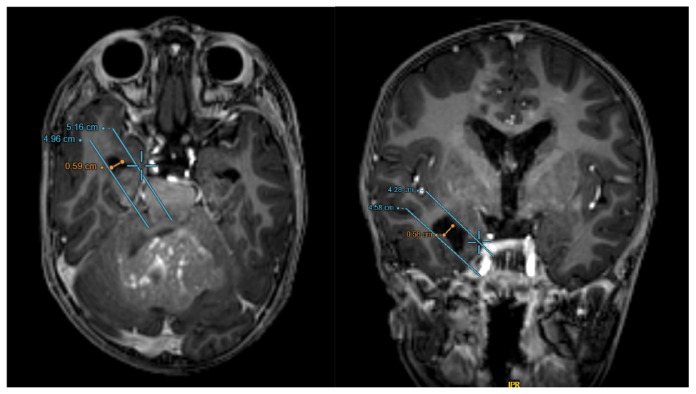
MRI Brain of the similar 3 years old child with grade 4 medulloblastoma as in the case in Figure 4
